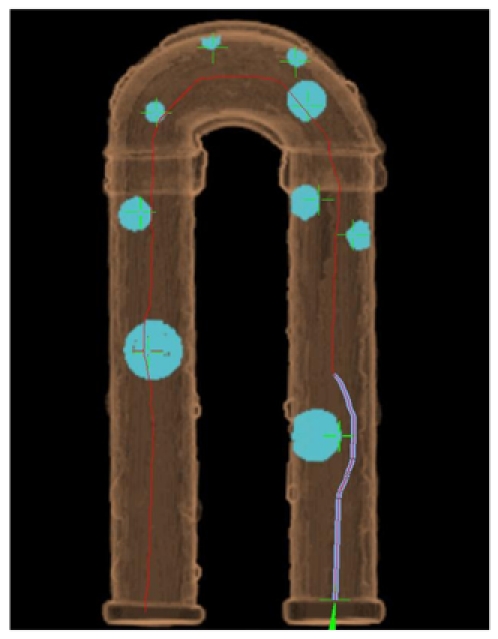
Placement of all polyps shown in a 3-dimensional overview image reconstructed from CT data.
